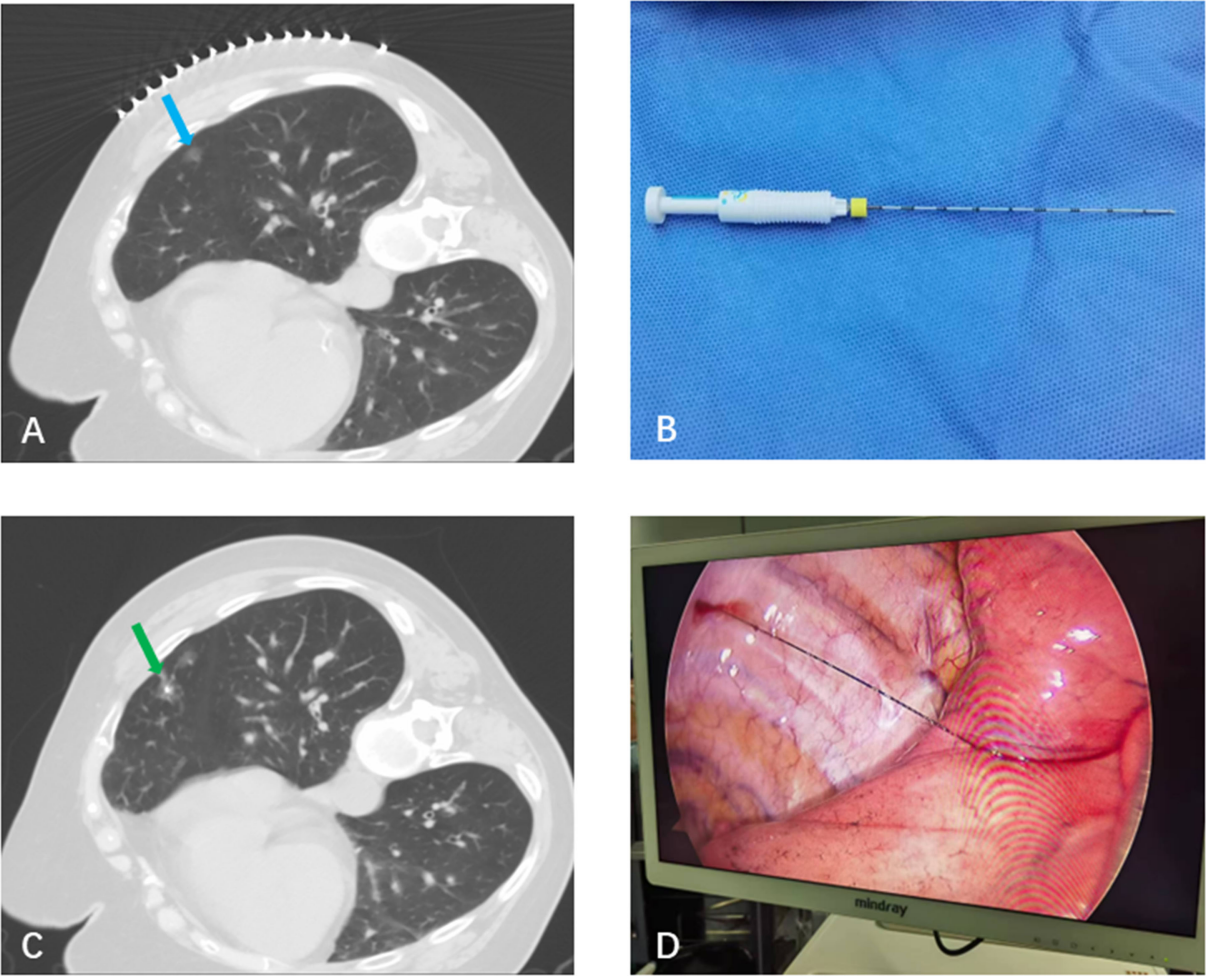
CT-guided localization and VATS resection in an 83-year-old male. (A) Initial CT scan showing a ground-glass nodule (blue arrow) in the right middle lobe. (B) The disposable soft hook-wire localization needle (Model FLS-20-100). (C) Confirmatory CT image to verify the anchor tip position; the green arrow indicates needle track hemorrhage. (D) Intraoperative view showing the hookwire located in the right middle lobe.
